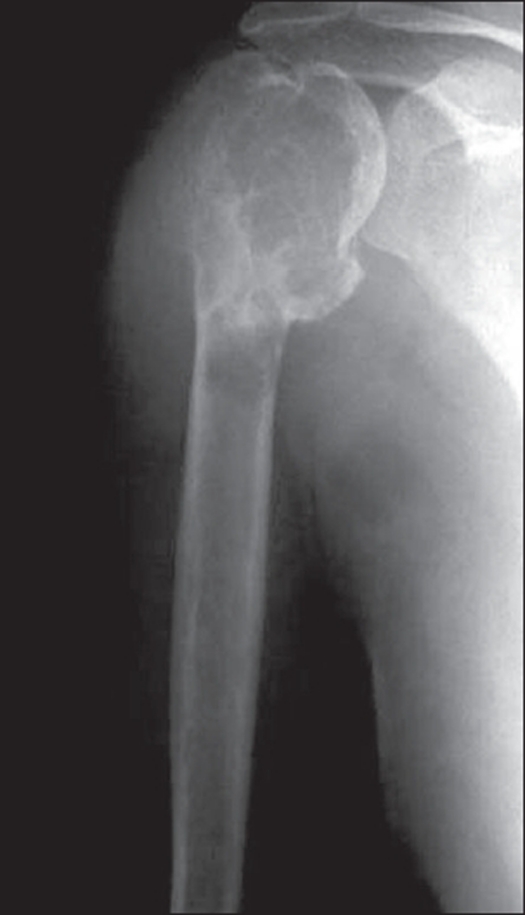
X-rays of GCT of proximal humerus showing extensive cortical destruction.
